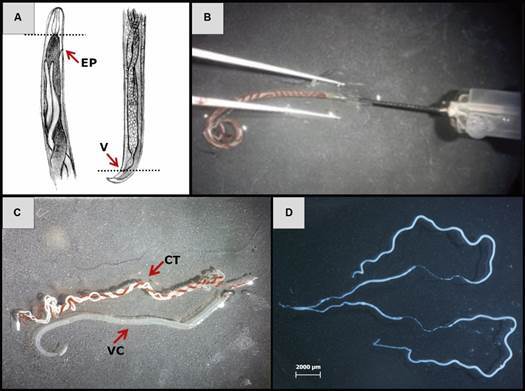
extraction of the reproductive system of adult females of *Angiostrongylus cantonensis*. A: identification of the sites for the sections (PE: excretory pore; TO: ovary terminations; V: vulva; An: anus); B: insertion of the needle into the anterior part of the worm; C: female after viscera extraction (CT = cuticle, and CV = visceral content); D: extracted female reproductive system.
